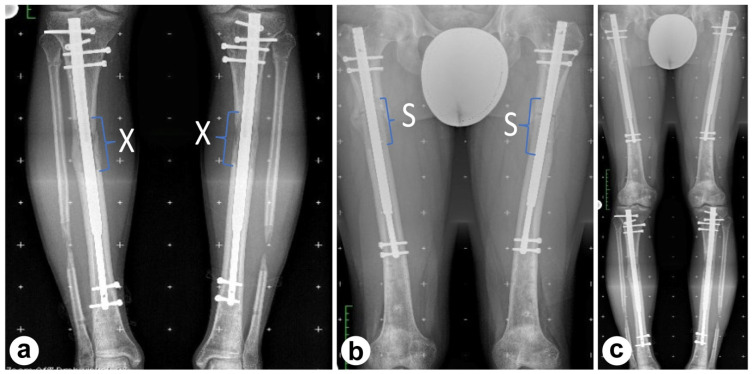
X-rays during third stage of procedure, X indicating the lengthening area: (a) X-ray upon completion of upper leg lengthening, (b) X-ray of both upper legs during cosmetic shortening procedure, S marking the shortened bone, and (c) long leg view of completed lower leg lengthening and completed upper leg lengthening.
